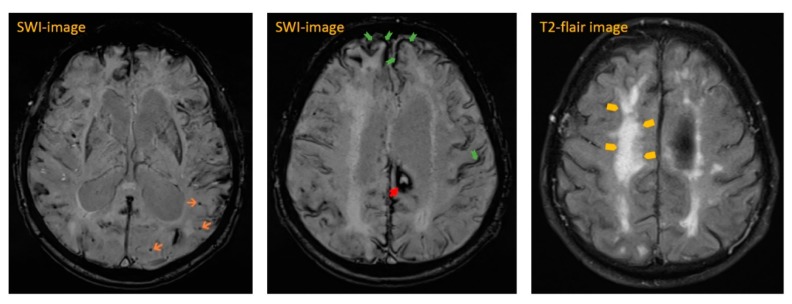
Suspicious findings of CAA-RI in patient’s MRI: micro- (orange colored arrows, left picture) and macrohemorrhage (red arrows, picture in the middle), superficial hemosiderosis (green arrows, picture in the middle) and T2-hyperintensities (yellow arrows, right picture) in susceptibility-weighted images (SWI) and T2-flair images.
